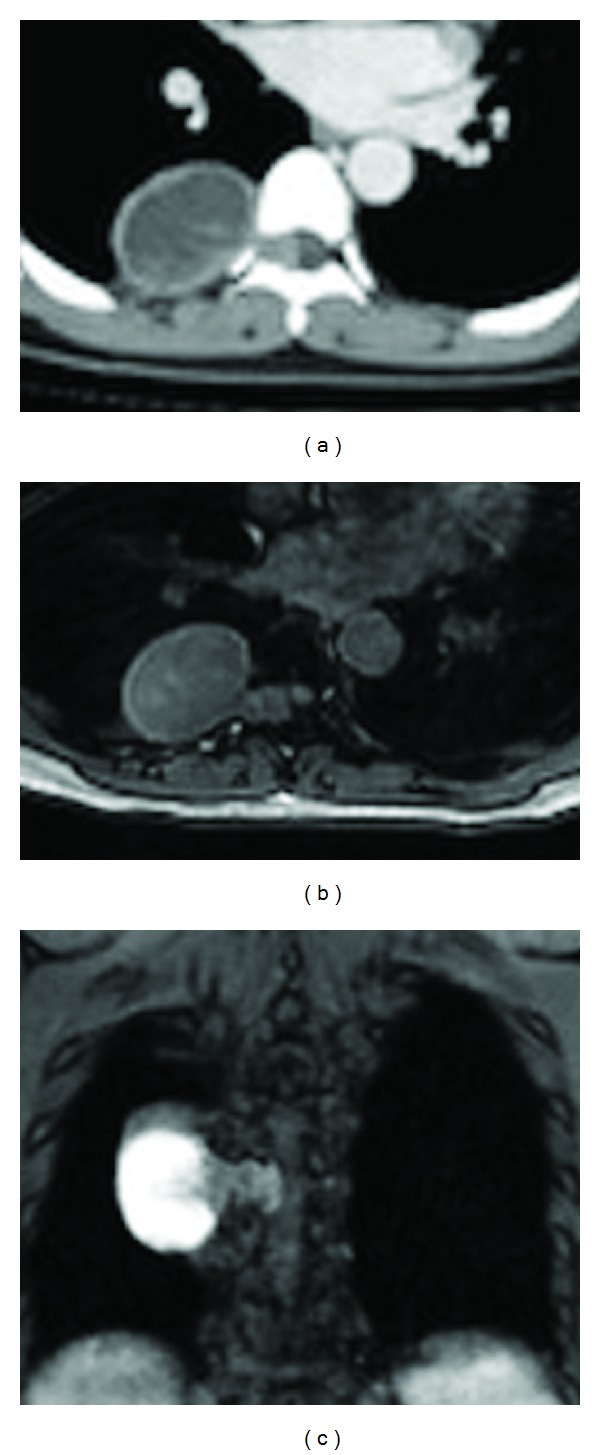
(a) Computed tomography (CT) scan showing a tumor occupying the spinal canal and extending into the paravertebral space through the right intervertebral foramen between Th7 and Th8. Axial (b) and coronal (c) T1-weighted magnetic resonance images with gadolinium enhancement, revealing a well-demarcated enhancing dumbbell-shaped tumor at Th7/8 on the right side. The tumor compresses the spinal cord anterolaterally towards the left side.
